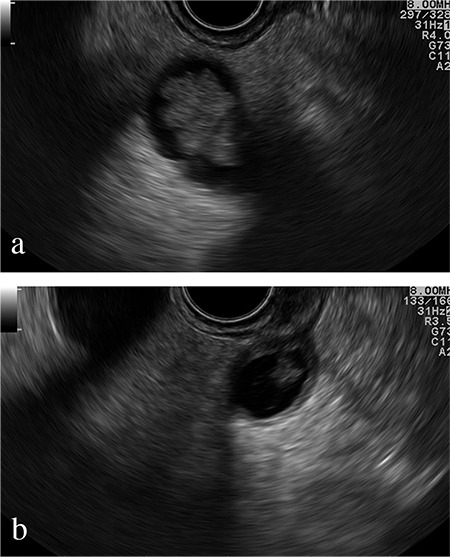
Transduodenal endoscopic ultrasound view of dilated common bile duct: lobulated soft tissue mass, “floating” in bile. Stalk is visible.
